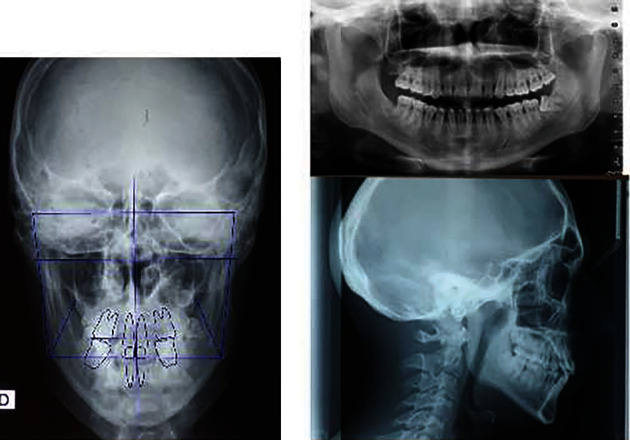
Post-treatment panoramic, antero-posterior, and cephalometric radiographs.
